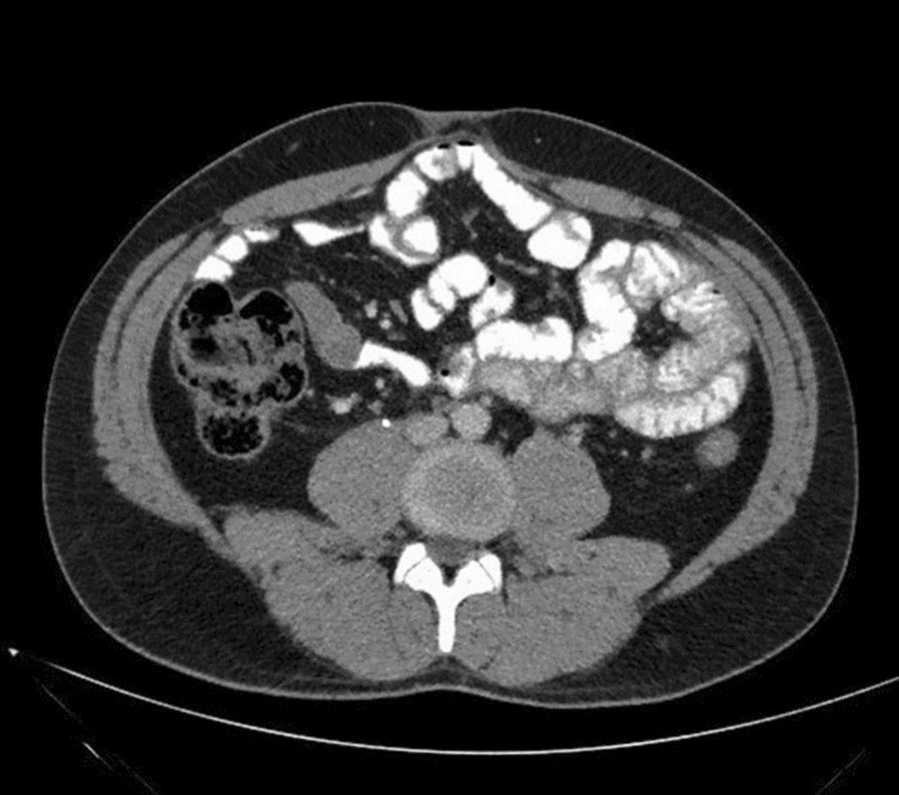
Abdominal contrast-enhanced CT 2 years after the operation showing no residual disease
